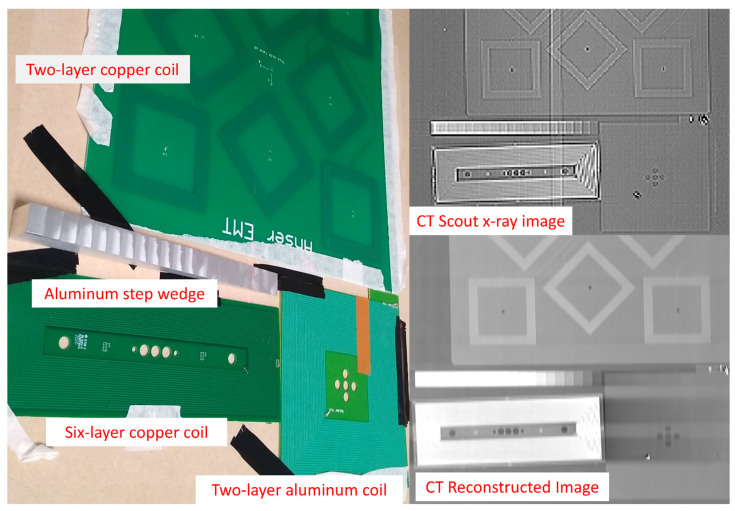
A similar assembly of test coils were scanned using the Toshiba Aquilion scanner. On the left of this image, the combination of the 2-layer aluminium coils, 2-layer copper coils, and a 6-layer coil design are positioned along with the aluminum step wedge. On the top right, we see the X-ray image from the CT scout X-ray image and bottom right we see an image resulting from the average, a 20 mm stack of reconstructed images.
